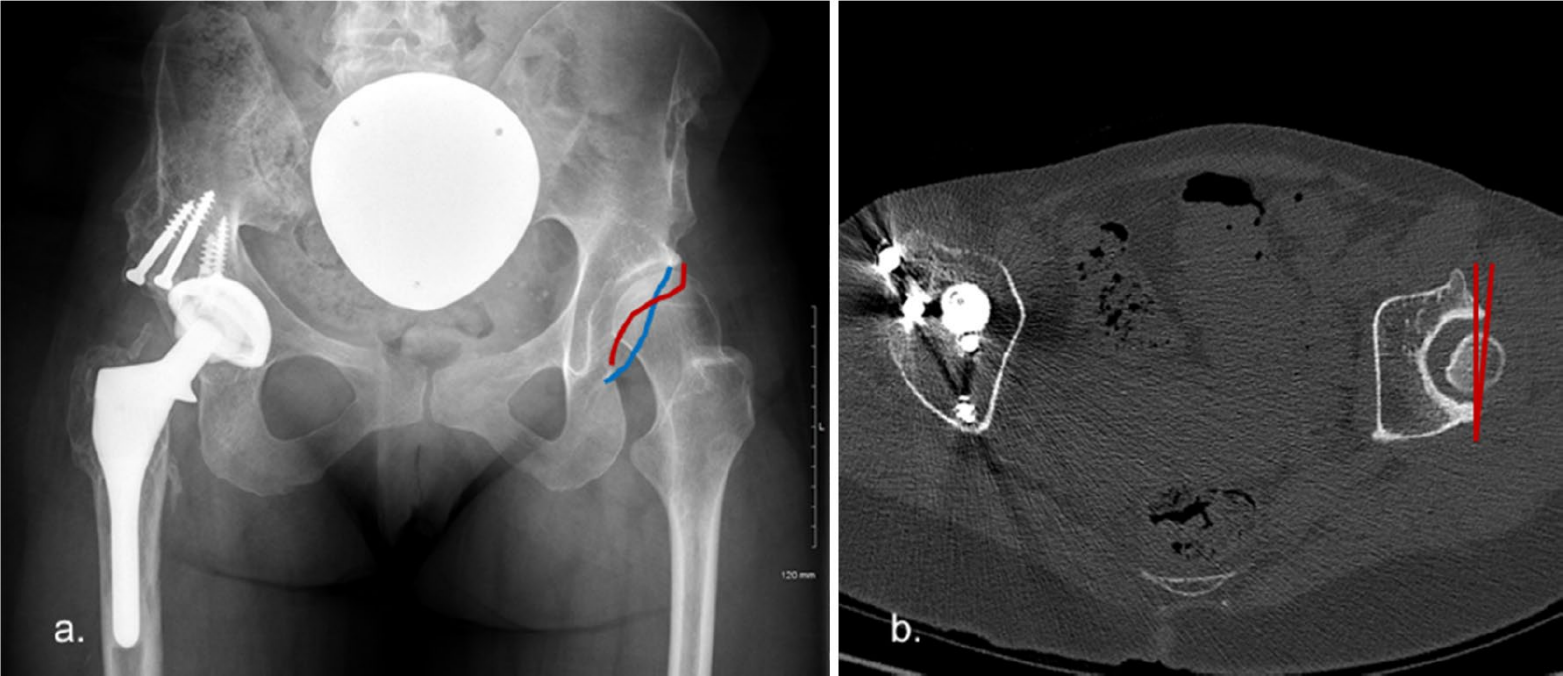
a AP radiograph pelvis. There is a crossover of the anterior and posterior walls of the left acetabulum, which represents acetabular retroversion. b CT axial image of the same patient which demonstrates left acetabular retroversion
